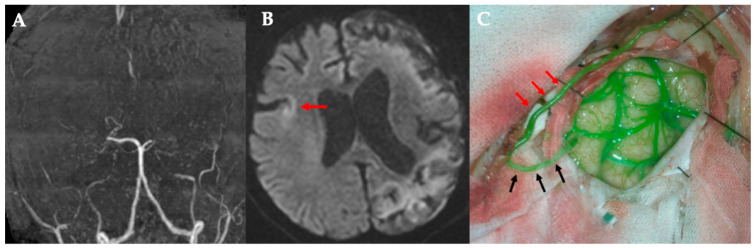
Case 2. The case of rapidly progressive MMD. (A) MRA. The main trunk of the internal carotid artery system has almost disappeared. (B) DWI. Right acute cerebral infarction occurred (red arrow) after the bypass surgery for the left cerebral hemisphere. (C) The side branch of the right STA parietal branch (black arrow) is anastomosed to M4 of the frontal lobe (black arrowhead), while blood flow is preserved in the main trunk (red arrow). MMD, moyamoya disease; MRA, magnetic resonance angiography; DWI, diffusion-weighted image; STA, superficial temporal artery.
